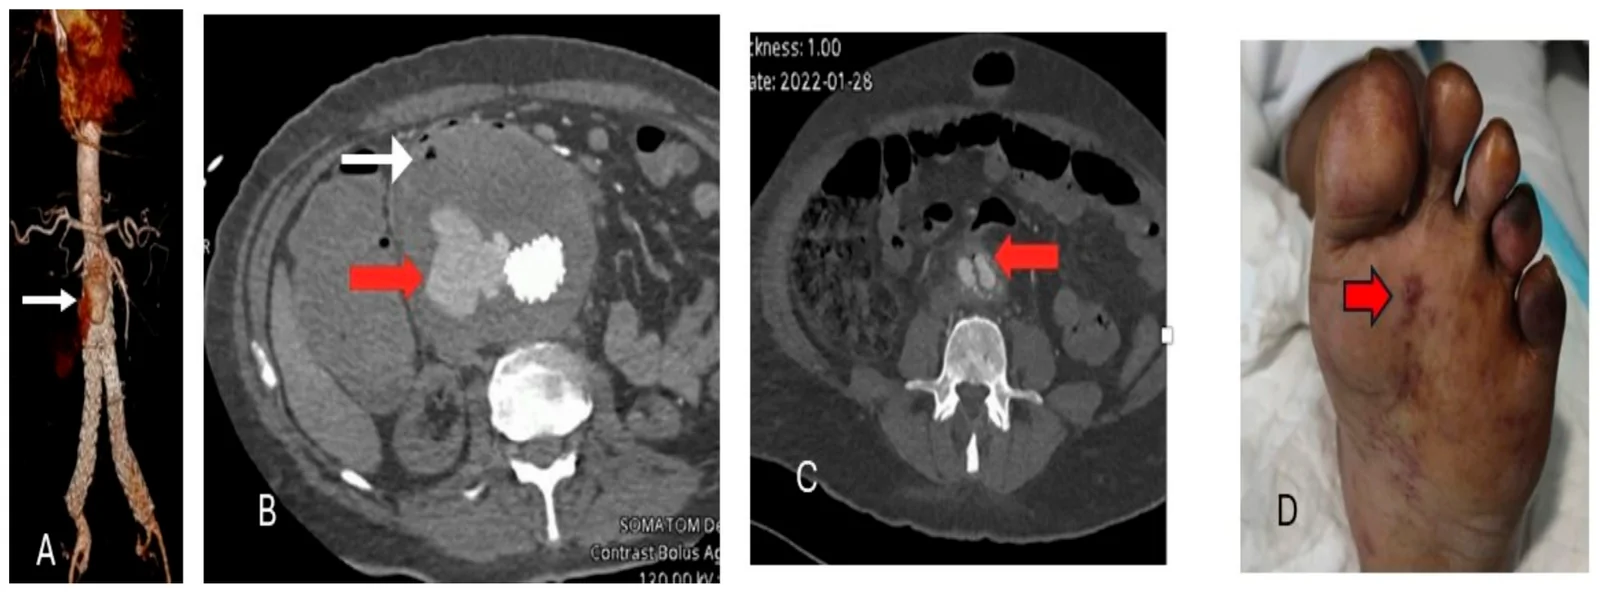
(Case 7): (A) Reconstructed CT scan with contrast, white arrow indicating large aneurysm with type one endoleak, also showing EVAR (Endovascular Aneurysm Repair) stent–graft. (B) CT scan with contrast (red arrow), indicating the large infrarenal abdominal aortic aneurysm with contrast outside the graft, indicating type I endoleak, and with an air bubble within the sac of the abdominal aneurysm, indicating AEF (white arrow). (C) Reconstructed CT scan with contrast (red arrow), indicating the distal part of the bifurcated graft and contrast; there is air around the graft, indicating infection. (D) A photo of the left foot showing ischemic changes (red arrow) due to septic emboli.
